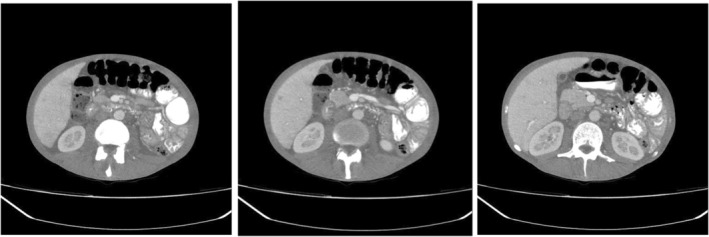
Abdominal CT scan. Hepatomegaly (hepatic span of 170 mm in midclavicular line) with heterogeneous enhancement and periportal edema were seen. An 8‐mm hypodense lesion with peripheral enhancement was observed in the anterior part of the sixth segment of the liver. Mild splenomegaly was seen (135 mm). Multiple mesenteric lymphadenopathies were also detected.
